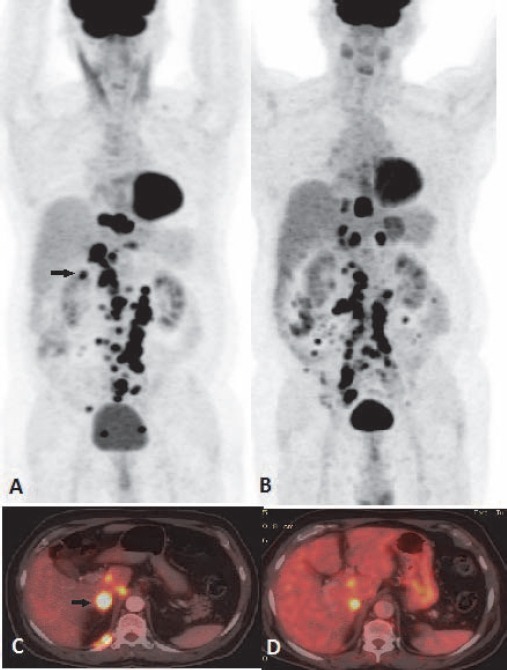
NHL - DLBCL involving adrenal gland: Nodule in right adrenal with increased FDG avidity (A and C). Post therapy scan shows decreased FDG avidity in suprarenal region (B and D)
